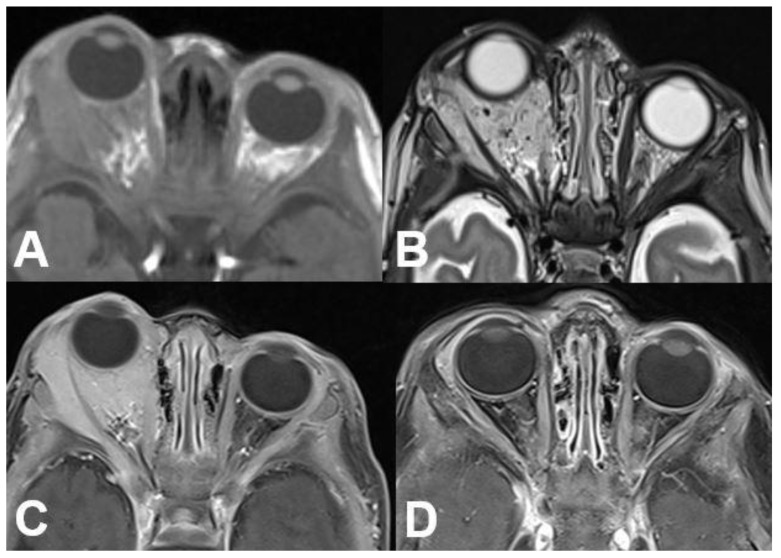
Capillary hemangioma.* MRI of a 12-week-old newborn female showing a tumor of the right orbit producing exophthalmos with slight isointense signal on T1-weighted images (A) compared to the orbit muscles and a hyperintense signal on T2-weighted images (B). The tumor shows homogenous enhancement in post-contrast images (C). After 11 months and therapy with propranolol, the tumor is not visible on exemplary post-contrast T1-weighted imaging (D).
